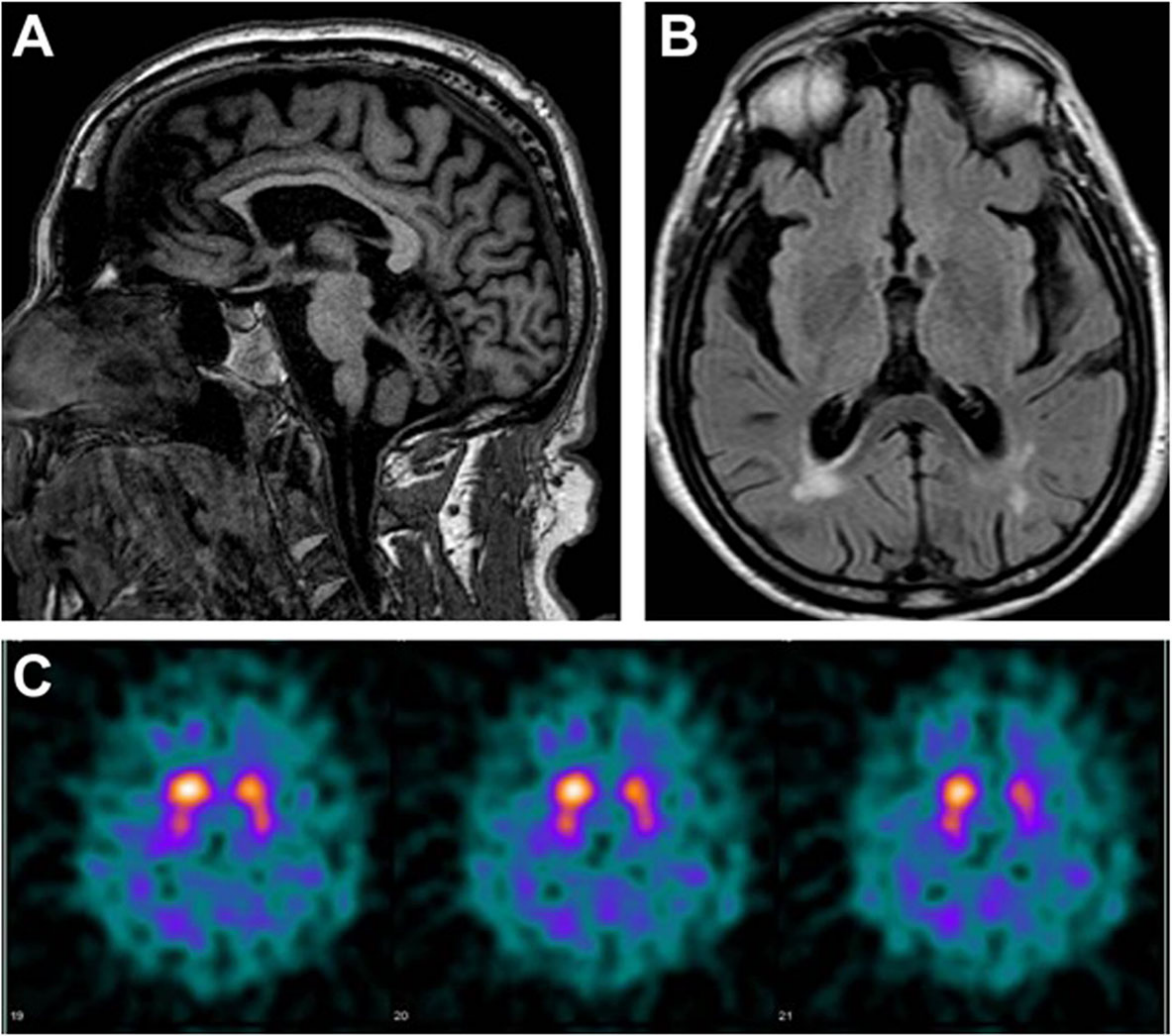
Brain magnetic resonance imaging showing: a marked ponto-cerebellar atrophy, and b signs of chronic cerebrovascular disease. Dopamine transporter imaging scan (c) showing bilaterally reduced putamen and caudate nucleus uptake
